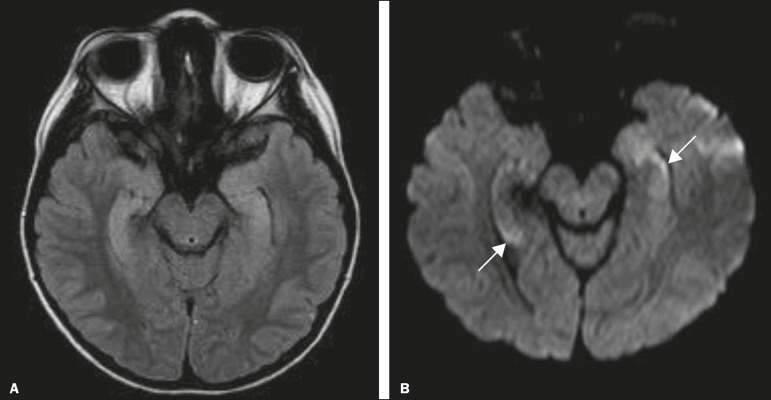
A 9-year-old female patient with behavioral changes, inversion of the sleep-wake cycle, and seizures. Tests for infectious and metabolic causes were negative. The cerebrospinal fluid tested positive for anti-GAD antibodies. Positron emission tomography/CT with 18F-fluorodeoxyglucose with no hypermetabolic changes suggestive of neoplastic involvement. Axial FLAIR-weighted MRI sequence and DWI (A and B, respectively) showing hyperintense signals in the mesial temporal regions with foci of restricted diffusion (arrows). A follow-up examination one year later showed a marked reduction in the volume of the hippocampal formations (image not shown).
